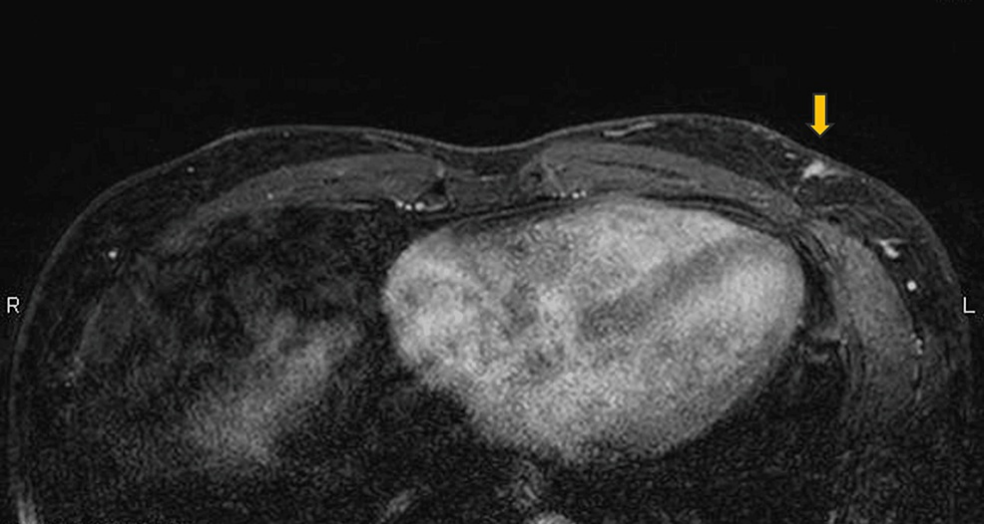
Dynamic contrast-enhanced MRI showing a 9 mm mass with mild progressive enhancement pattern (type I curves). MRI, magnetic resonance imaging
